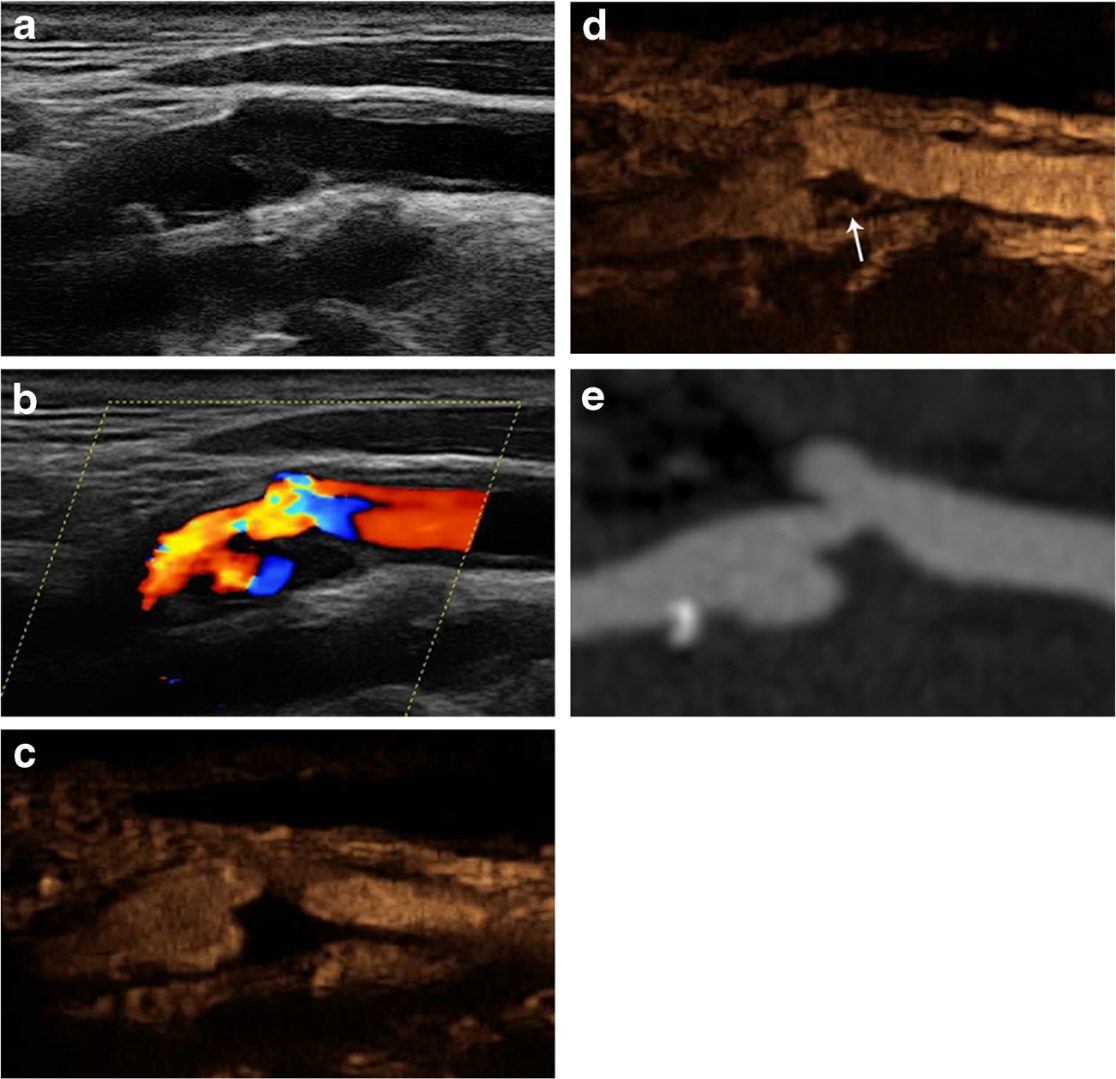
Imaging findings of an ulcerated carotid plaque with intraplaque neovascularisation. B-mode image (a) identified the presence of an anechoic cavity possibly representing an ulceration. Colour Doppler image (b) showing flow reversal within the ulcer cavity. CEUS (c) a few seconds after the intravenous administration of microbubbles confirms the presence of ulceration while the plaque appears anechoic. Delayed CEUS image (d) revealed the presence of moving microbubbles within the plaque and near its adventitial side representing intraplaque neovascularisation (*arrow*). MDCTA image (e) showing the ulcerated plaque in correlation with ultrasonographic techniques
